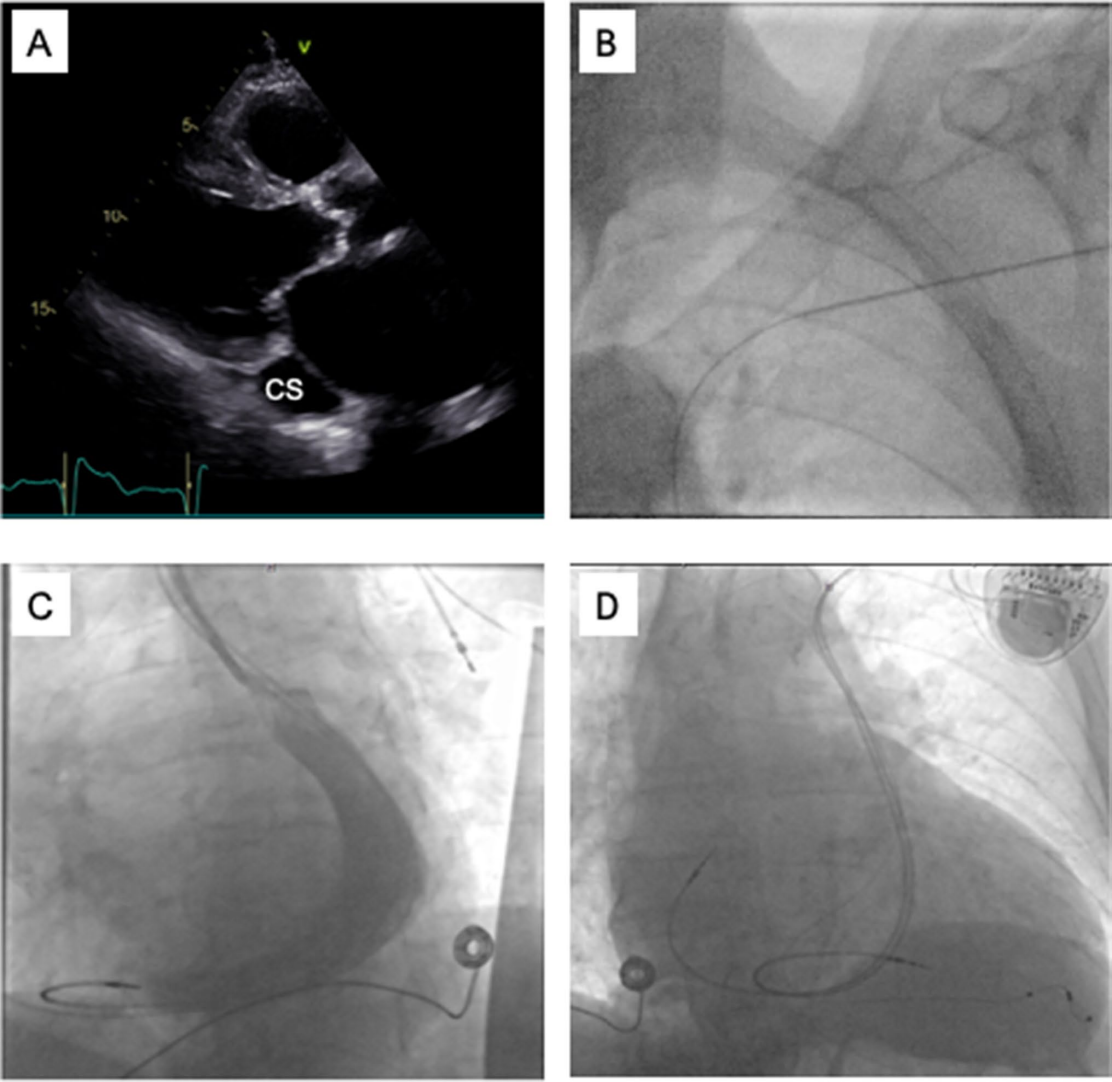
Case # 1: transthoracic echocardiography showing a dilated coronary sinus (CS) in parasternal long axis view (A). Wire passing through PLSVC (B). Non-selective antegrade CS angiography showing dilated CS and the right ventricular lead, left anterior oblique view (C). Fluoroscopic overview after implantation of all three leads and CRT-P device, postero-anterior view (D)
